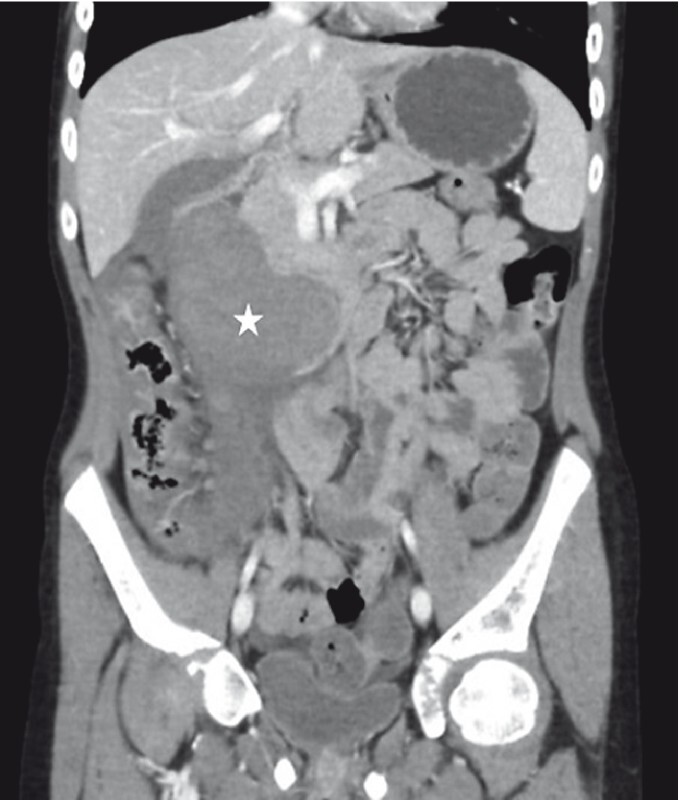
Computed tomography scan image (coronal view) showing a large intramural duodenal hematoma (*).
